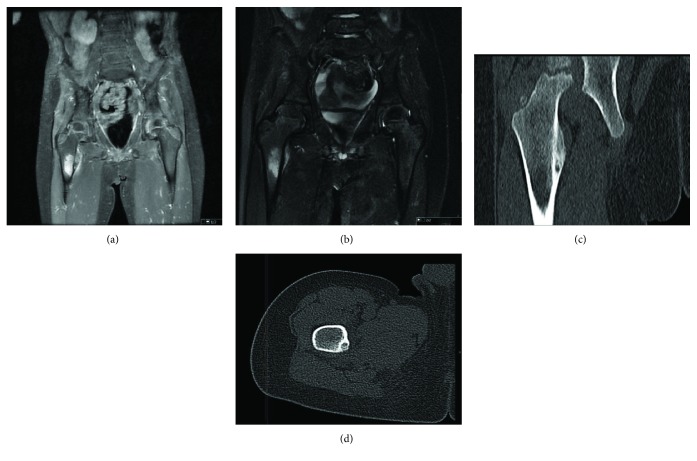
(a, b) MRI with diffuse edema both on T1 with gadolinium uptake and STIR, on the lesion of the lesser trochanter. (c, d) CT scan with the nidus in the lesser trochanter.
